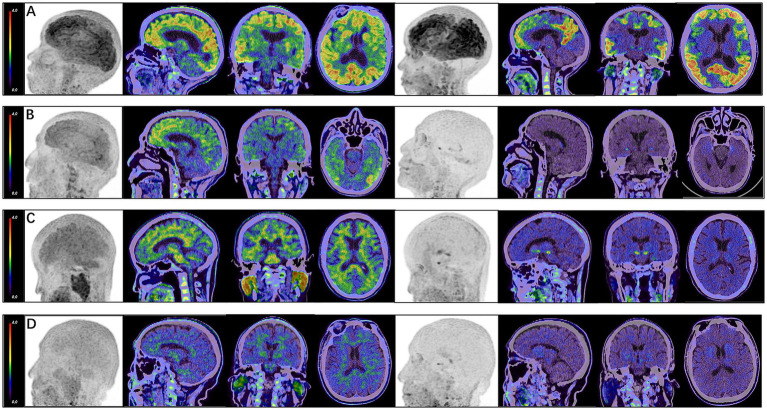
Representative images of ^18^F-AV45 and ^18^F-AV1451 PET/CT in patients with AD, MCI, and HC. For each subject, images are shown from left to right: sagittal ^18^F-AV45 PET MIP, sagittal PET/CT fusion, coronal PET/CT fusion, axial PET/CT fusion, followed by the same series for ^18^F-AV1451. (A) A 54-year-old female with AD showing extensive diffuse A*β* and tau deposition. (B) A 72-year-old male with MCI showing marked diffuse Aβ deposition with abnormal tau in bilateral temporal lobes. (C) A 69-year-old female with MCI showing mild Aβ and tau deposition in bilateral hippocampi. (D) A 72-year-old male HC with no significant Aβ or tau deposition.
